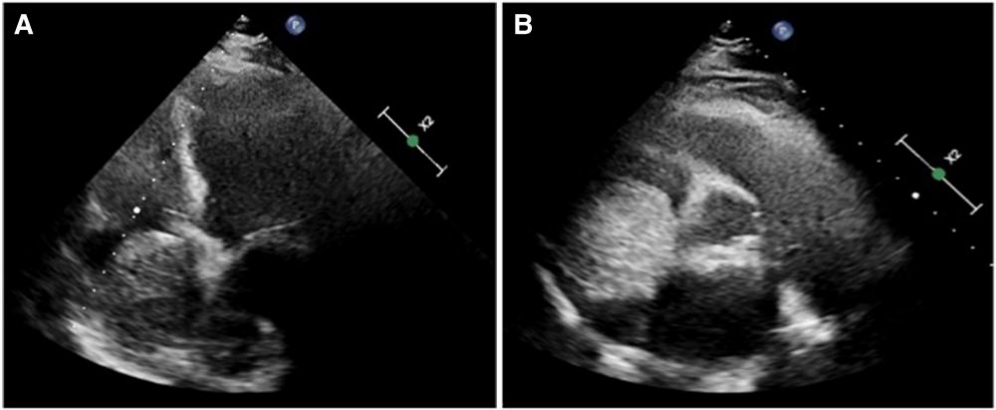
Transthoracic echocardiography—tumour in right atrium. (*A*) Apical four-chamber view; (*B*) parasternal short axis view at the aortic level). Own study.
